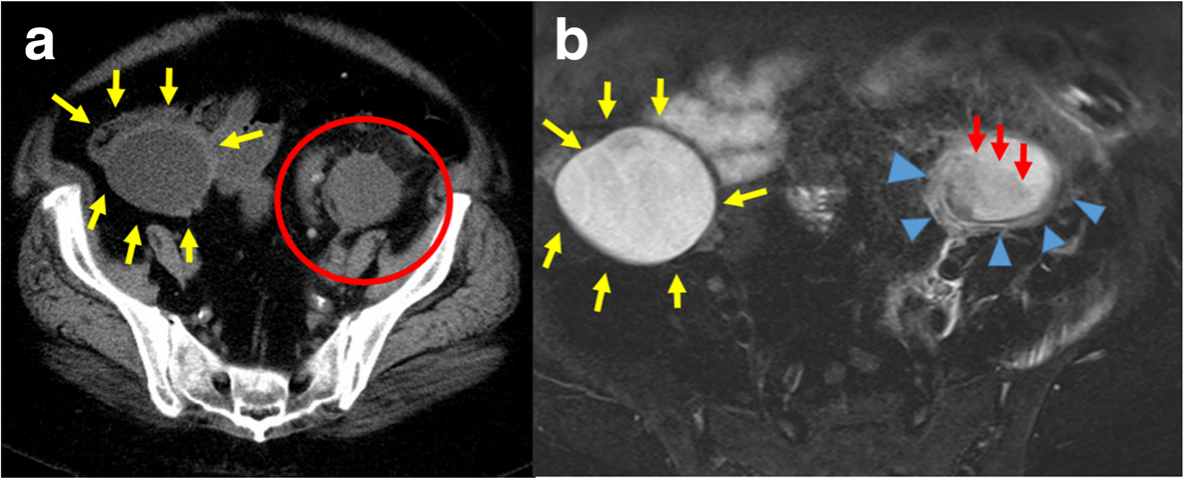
Computed tomographic and magnetic resonance imaging findings on admission. a Computed tomography scan of the abdomen and pelvis, showing a 38 × 44-mm tumor surrounded by dense adipose tissue (*red circle*) in the left adnexa. The previously noted right ovarian abscess also is seen (*yellow arrows*). b Coronal T2-weighted contrast-enhanced magnetic resonance image of the pelvis, showing edematous change (*red arrows*) surrounded by liquid formation (*blue arrowheads*) in the left adnexa. The previously noted right ovarian abscess also is seen (*yellow arrows*)
